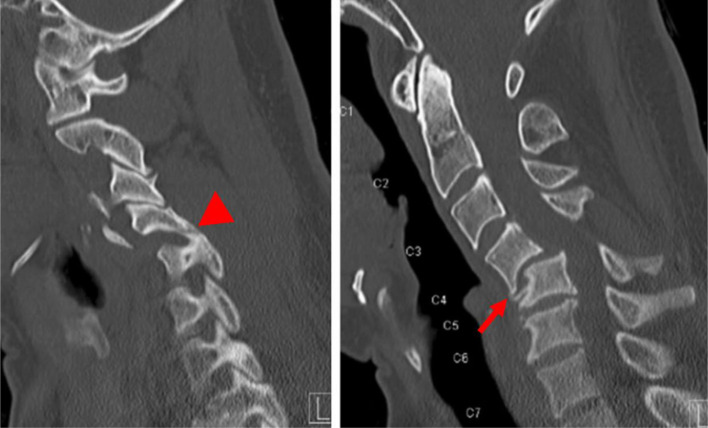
C-spine CT-scan sagittal slices image demonstrating a neglected C6–C7 facet dislocation. Note the osteocartilaginous tissues bridging C6 and C7 (red arrow) and locking of facets (red arrowhead).
